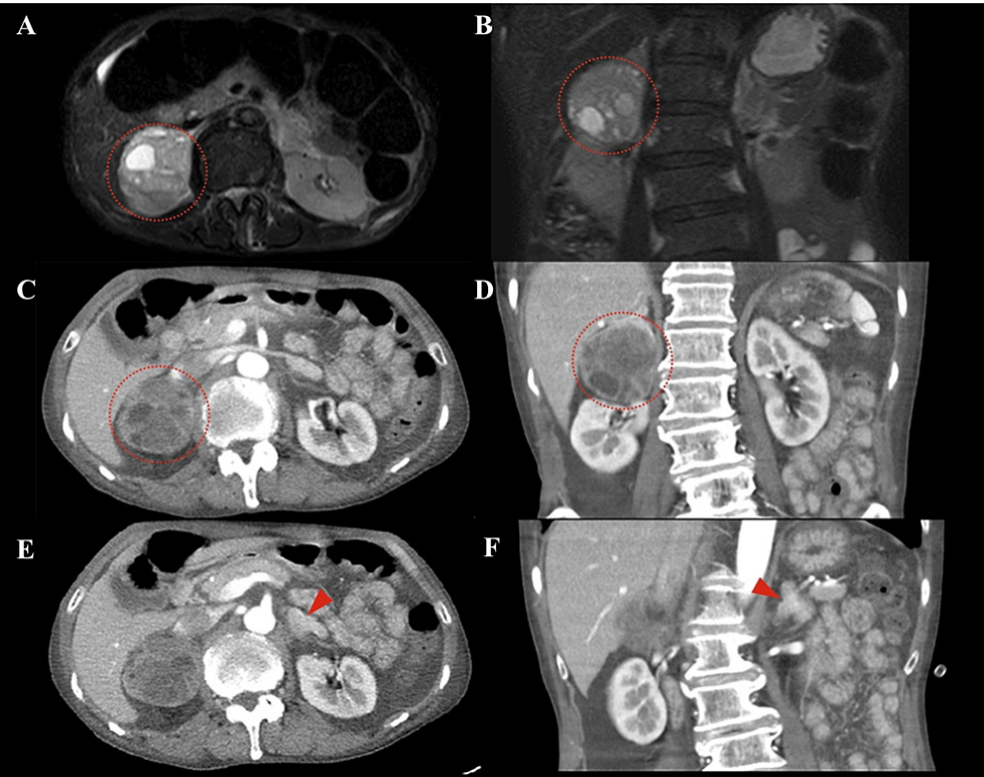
Representative images acquired during the patient’s admission prior to adrenalectomy The images include axial (A) and coronary (B) views from the T2-weighted MRI, along with axial (C, E) and coronal (D, G) views of the enhanced CT scan. Dotted circles and arrowheads indicate the presence of a pheochromocytoma in the right adrenal gland and an enlarged left adrenal gland, respectively.
